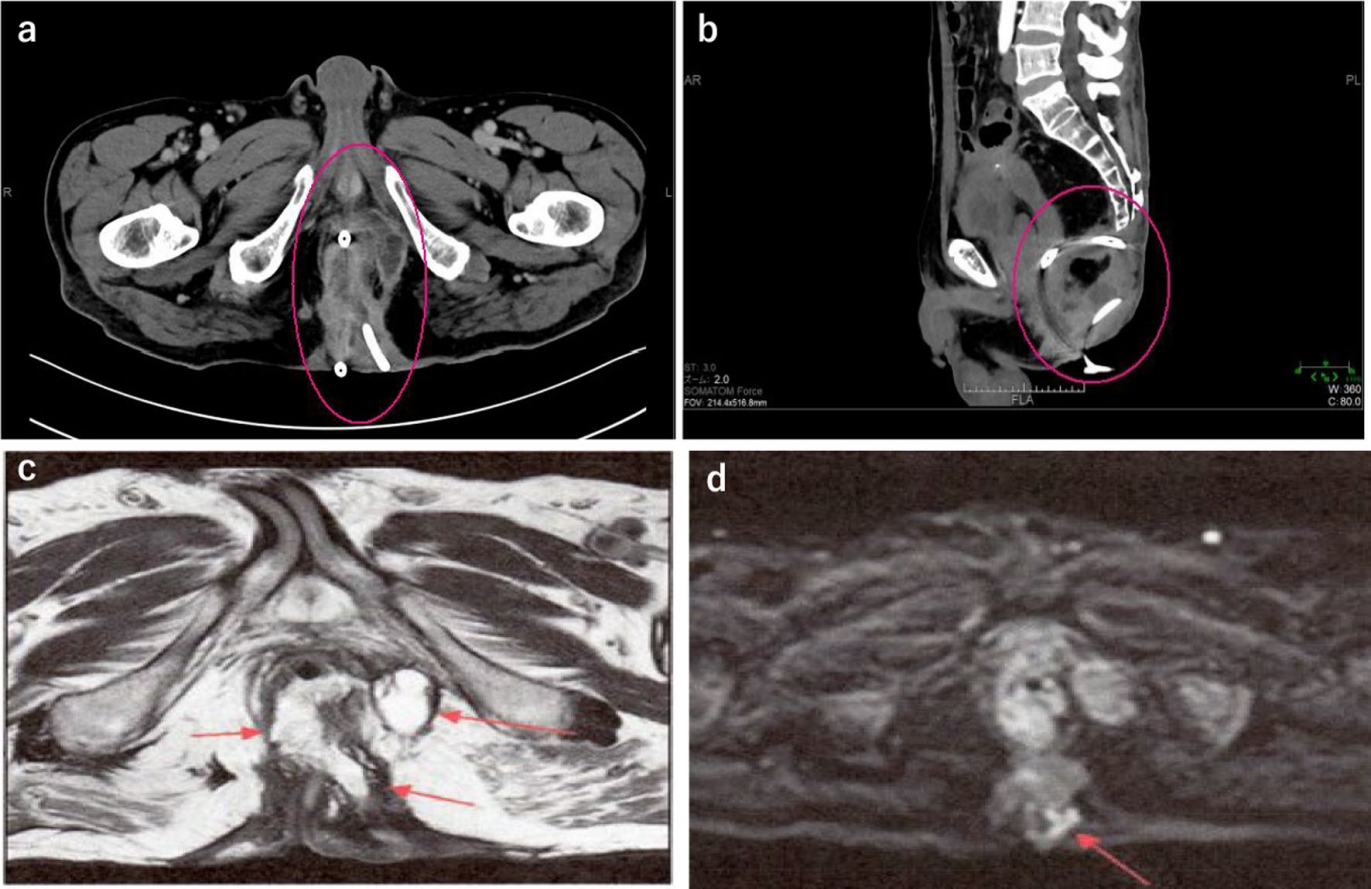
Abdominal CT (a, b) and MRI (c, d) shows the presence of anal fistula (circle and arrow) to the lower sacrum from the anus
